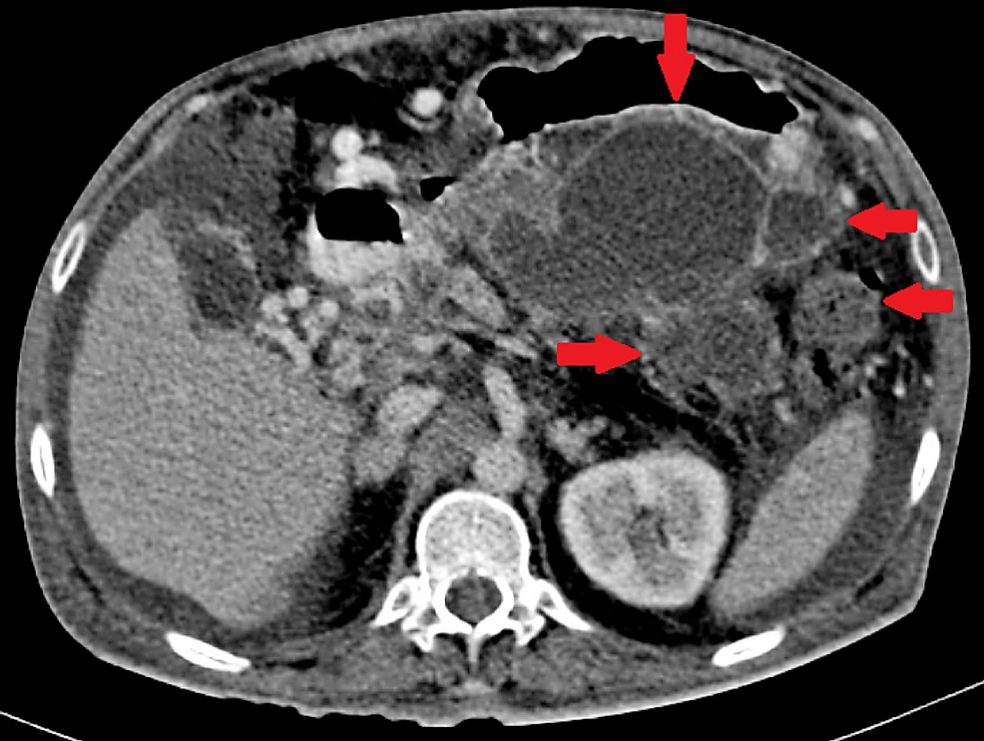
Contrast-enhanced computed tomography of the abdomen showing multiple pancreatic pseudocysts in the abdomen
